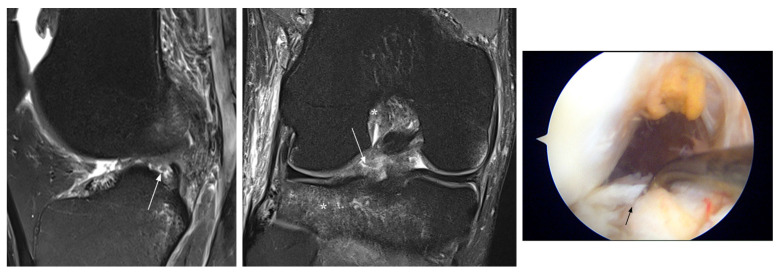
Interpretation false-negative MRI diagnosis (patient 18). MRI shows a tear at the posterolateral meniscal root that was missed at prospective reading and confirmed by arthroscopy (arrows). Also note lateral bone contusions and acute ACL injury (*).
